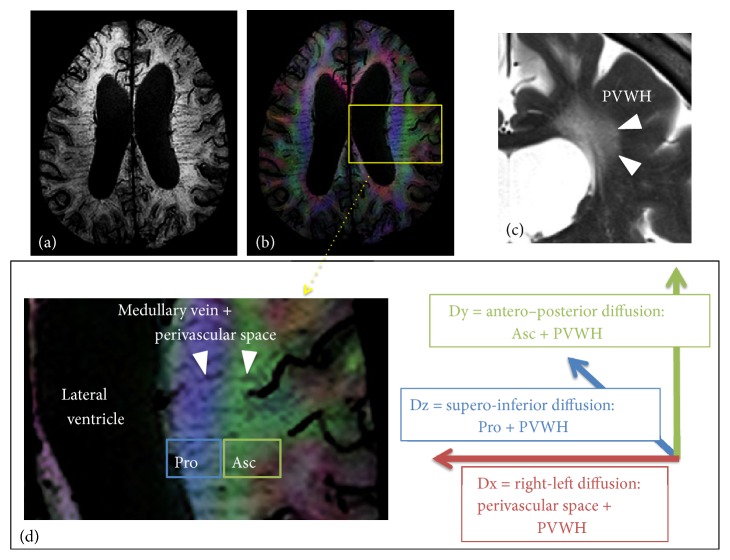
(a) Susceptibility-weighted image (SWI) shows the medullary veins running in the periventricular white matter. (b) Fusion of SWI and color-coded FA map. The periventricular white matter is divided into two regions; medial blue and lateral green areas, which correspond to the projection and association fibers, respectively. (c) In cases with ventriculomegaly, PVWH is often observed on T2-weighted image. (d) The medullary vein runs through the association and projection fiber areas (Pro and Asc). We hypothesized Dx reflects perivascular space of the deep white matter vessels and PVWH. Therefore, Dx partially include the glymphatic system. Dy and Dx are supposed to reflect the association fiber plus PVWH and the projection fiber plus PVWH, respectively.
